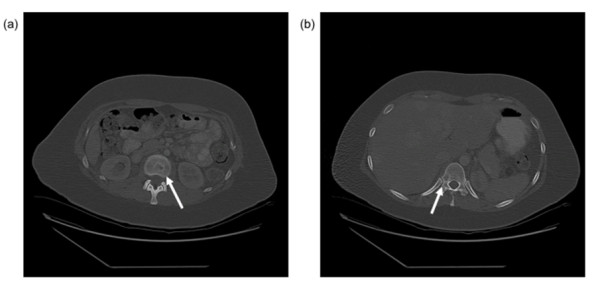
Representative magnetic resonance image revealing bone metastasis indicated by the white arrow.
